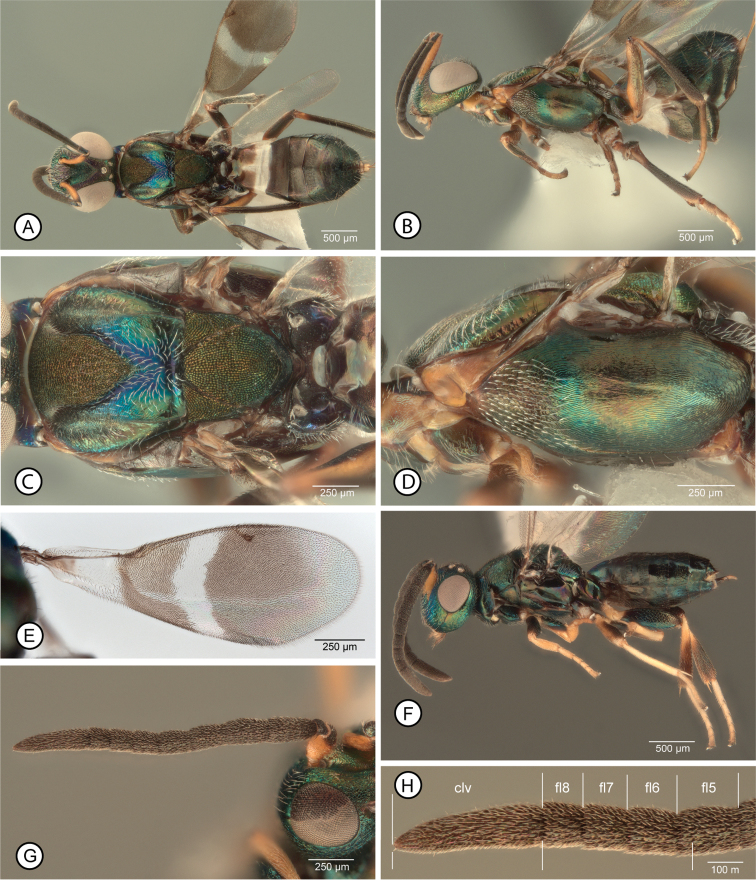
Anastatusfulloi*A–E female: A dorsal habitus (13) B lateral habitus (12) C dorsal mesosoma (13) D lateral mesosoma (12) E fore wing (14). F–H male: F lateral habitus (22) G antenna (32) H clava and apical three funiculars (32) (three lower bars indicate length of clava compared to combined length of apical funiculars). Abbreviations: clv = clava, flx = flagellomere number.
